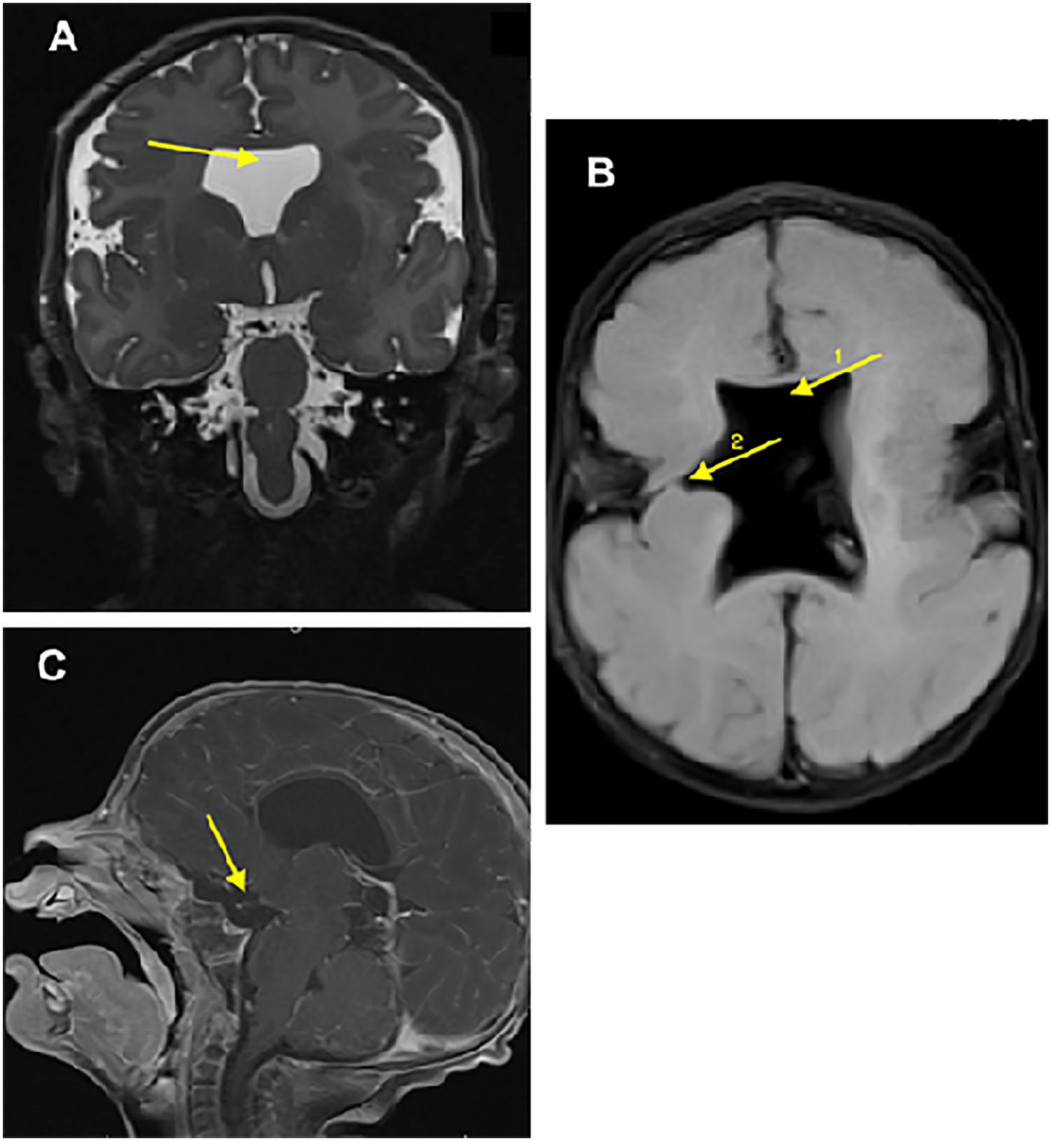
T2-weighted brain magnetic resonance imaging (MRI) images showing an absent septum
